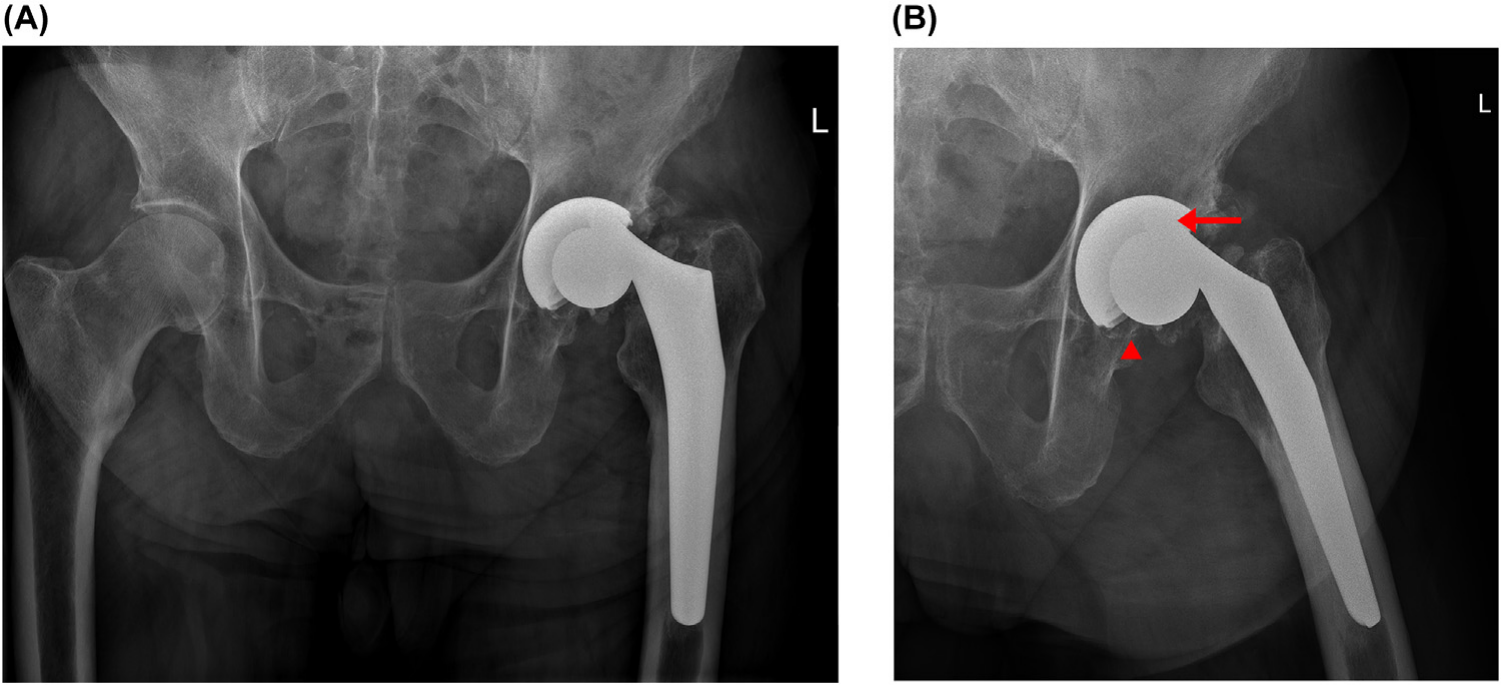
Radiographs of the (A) pelvis and (B) left hip demonstrate a metal-on-polyethylene design left total hip arthroplasty. There is dissociation of the prosthetic femoral head from the femoral stem. The trunnion is tapered and is superolateral relative to the femoral head, projecting into the region of the polyethylene liner (arrow). The femoral head component remains located within the acetabular component. Metallic debris is visible (arrowhead). Nonbridging heterotopic ossification is present at the superolateral aspect of the hip.
